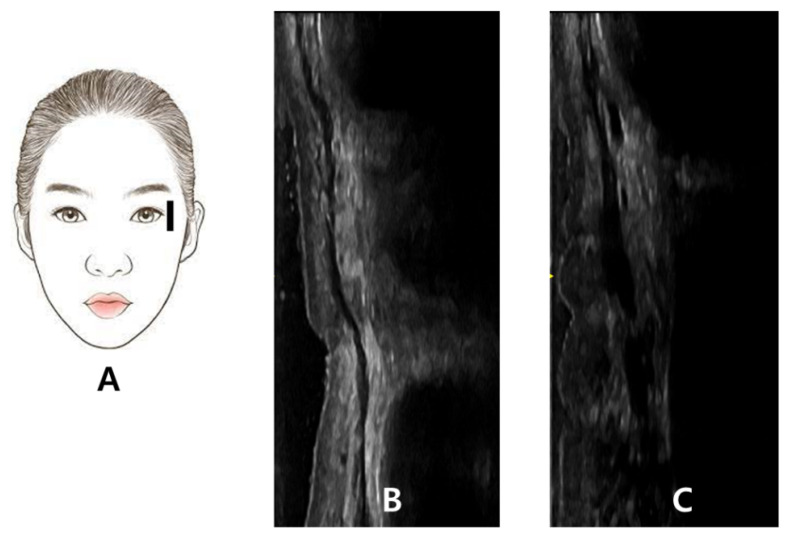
The probe of the ultrasonography has been placed longitudinally at lateral orbital rim at the level of lateral canthus (A). The thin hypoechoic orbicularis oculi muscle observed in ultrasonography during resting state (B). The thick hypoechoic orbicularis oculi muscle is observed in contracted state when asked to smile (C).
